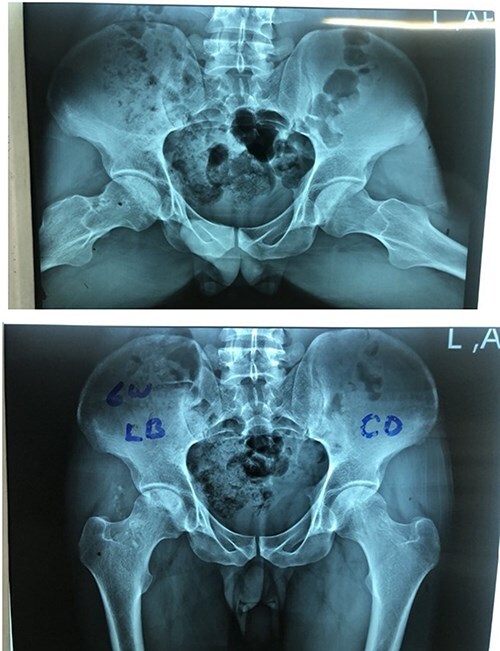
The 6 week postoperative radiographs comparing lightbulb procedure in the right side and core decompression in the left side.
